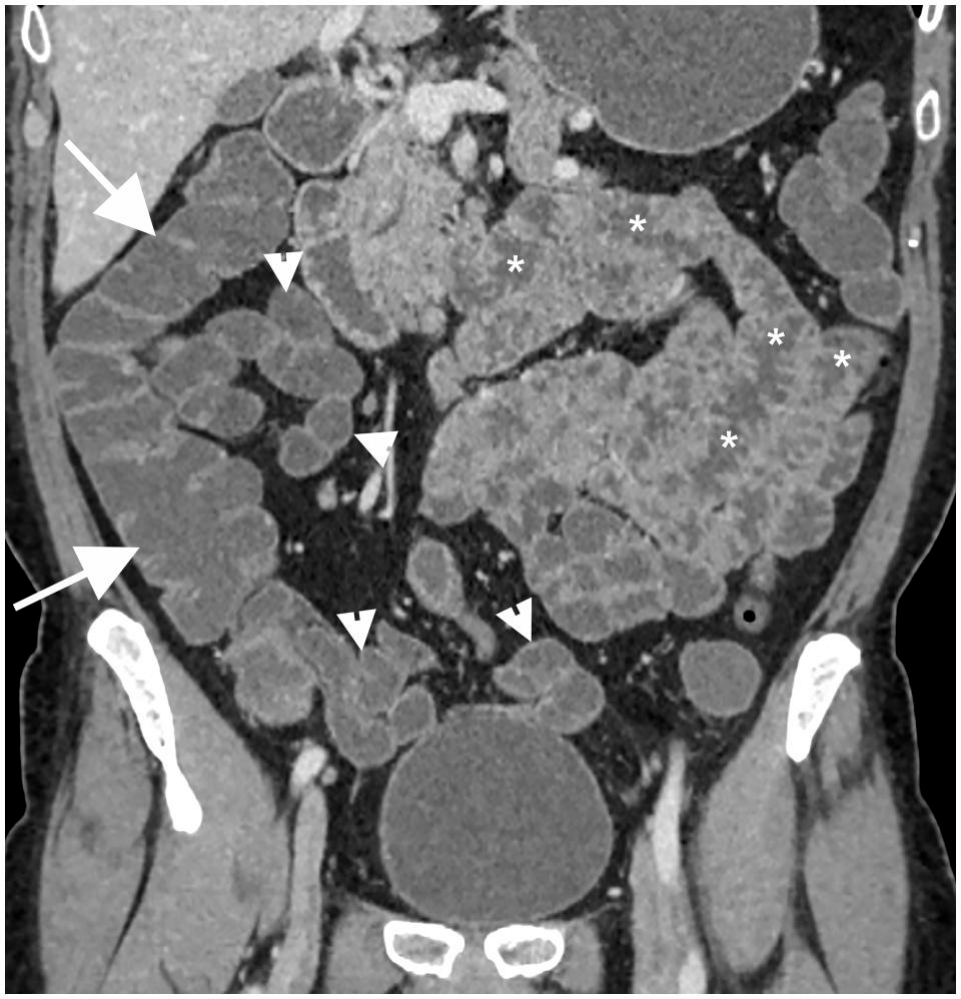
CT-Enterography: neutral contrast agent administered by mouth allows a variable degree of colic distension (white arrows) as seen in this coronal CT image; a good distension of some ileal loops (white arrowheads) has been achieved and acceptable distension of jejunal loops (asterisks) is also evident.
